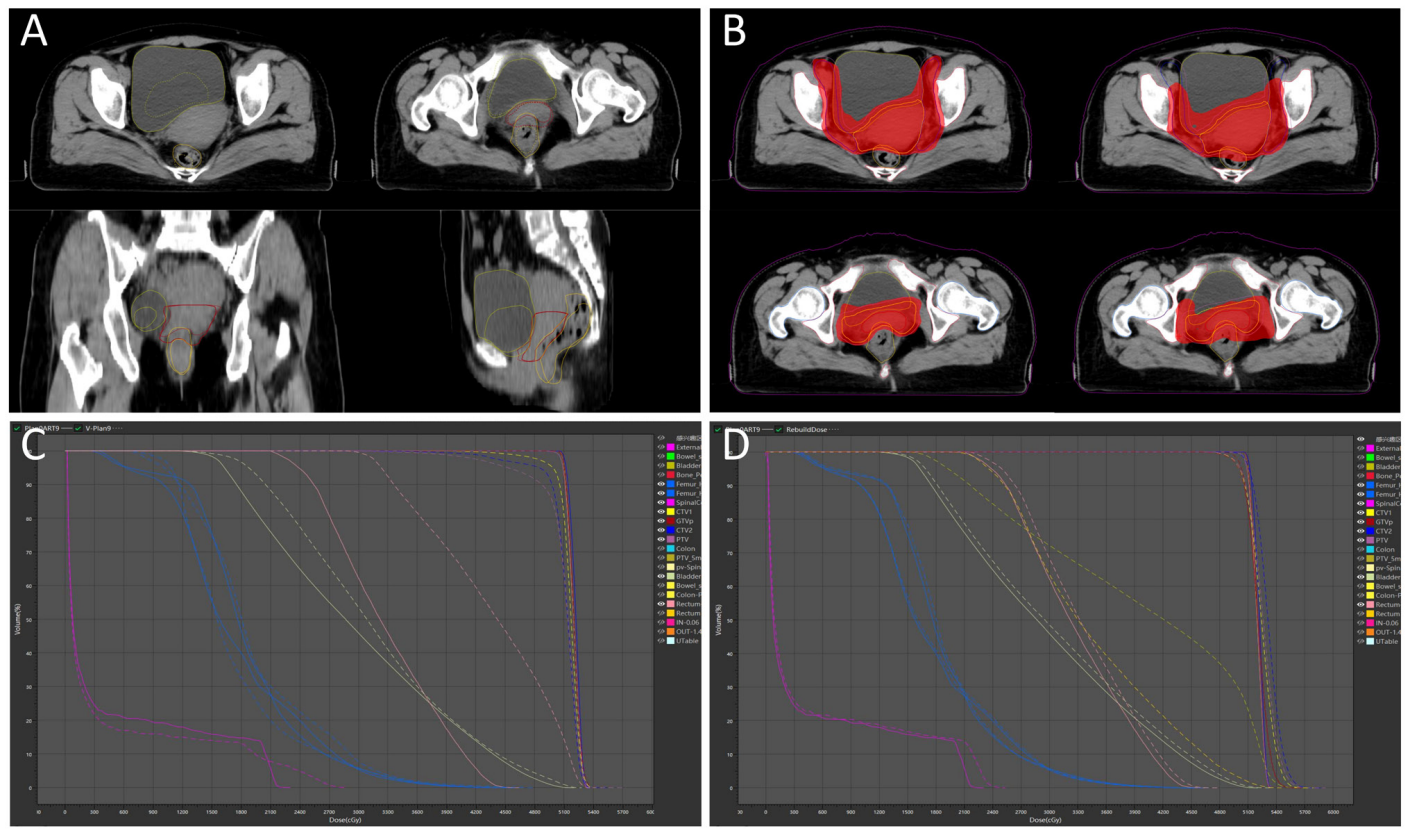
Effect of rectal, bladder and lesion volume changes on dose distribution. (A) The changes of volume in bladder, rectum and GTVp between the images of 9th fraction and positioning computed tomography. (B) Difference in dose distribution between the 9th fractional ART-Plan and IGRT-Plan. (C) The DVH comparison of the 9th fractional ART-Plan and IGRT-Plan. (D) The DVH comparison between 9th fractional ART-Plan and *in vivo* dose verification of 3D reconstruction. GTVp, primary gross tumor volume; DVH, dose-volume histogram; IGRT, image-guided radiotherapy; ART, adaptive radiotherapy.
